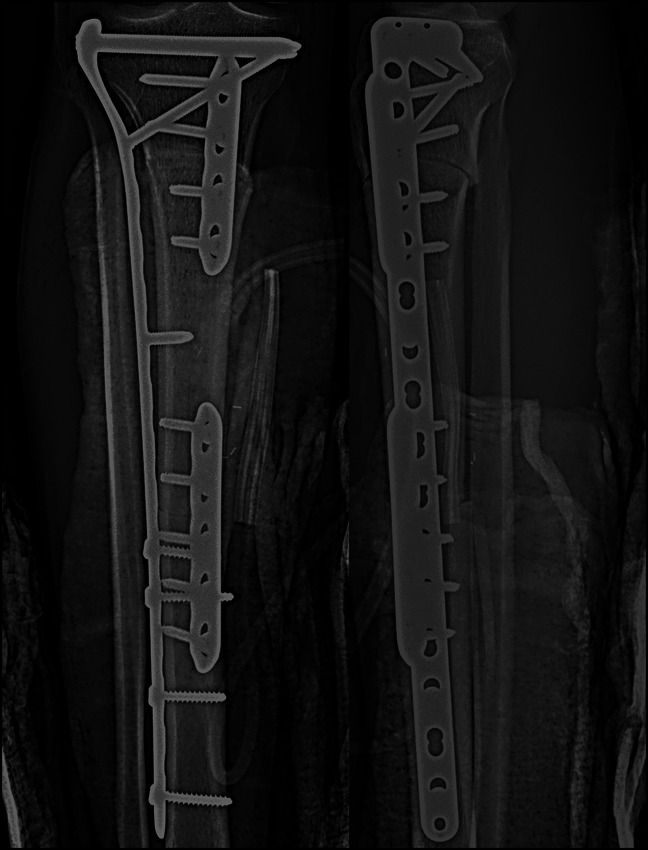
Radiograph of 27-year-old man demonstrating hemispherical reaming at metadiaphysis and mid-diaphysis graft-host interface (case 1: AP and lateral radiographs after intercalary allograft reconstruction on postoperative day zero). Interfaces are compressed with low-contact dynamic compression plates with bridge plate expansion of entire construct.
